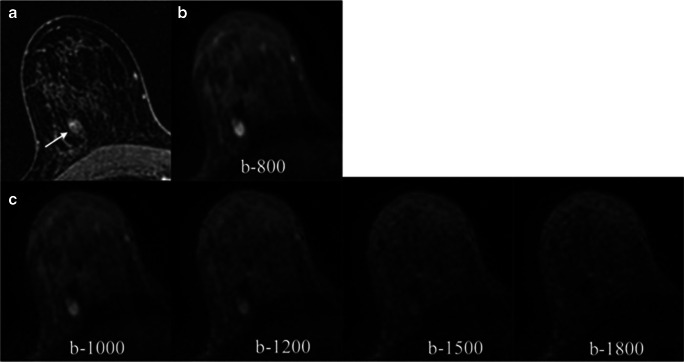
Axial contrast-enhanced (a), acquired DWI (b), and synthetic DWI (c) images of a patient with a 10-mm biopsy-proven fibroadenoma in the third posterior depth of the right breast. This benign lesion is less conspicuous at higher b-values being barely visible at b-1500 and b-1800 s/mm^2^. ADC mean value for this lesion was 1850 × 10^−6^ mm^2^/s. This lesion was correctly categorized as non-suspicious in both DWI and DCE-MRI. DWI, diffusion-weighted imaging; ADC, apparent diffusion coefficient; DCE-MRI, dynamic contrast-enhanced magnetic resonance imaging
